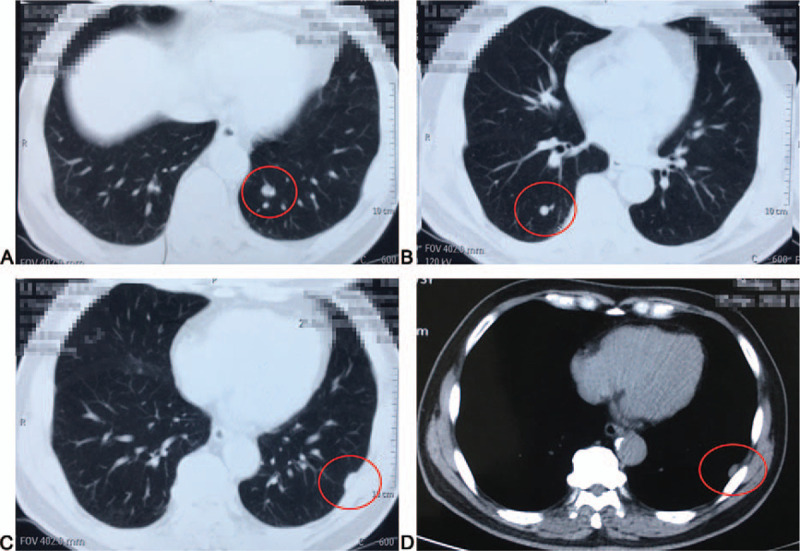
(A–D) Pulmonary computed tomography (CT) suggested bilateral lung and left pleural (red circle) metastases after a dacarbazine/interleukin-2 regimen for 4 cycles.
